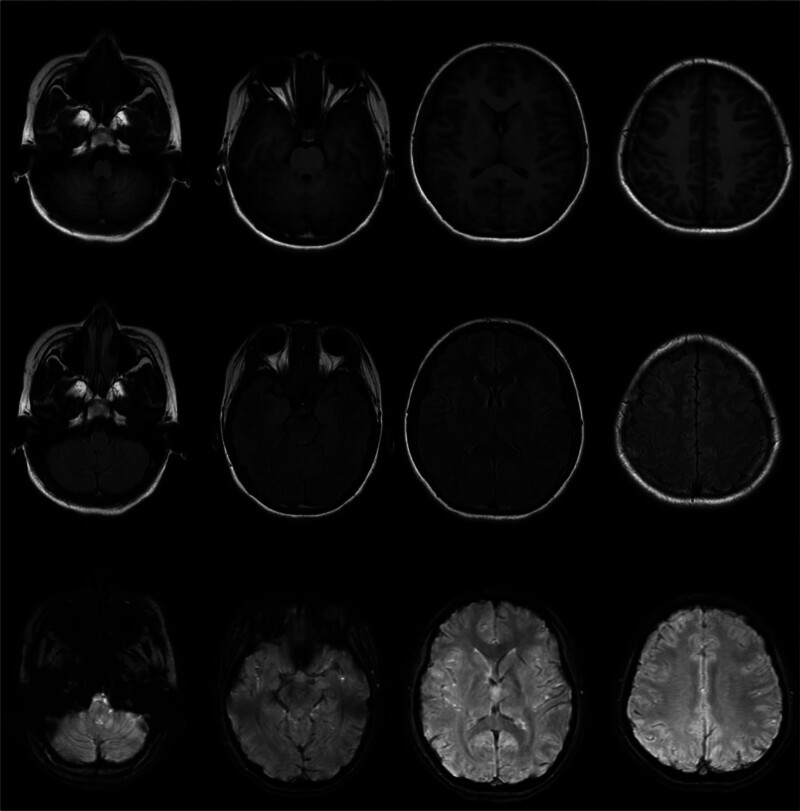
Magnetic resonance imaging (MRI).
